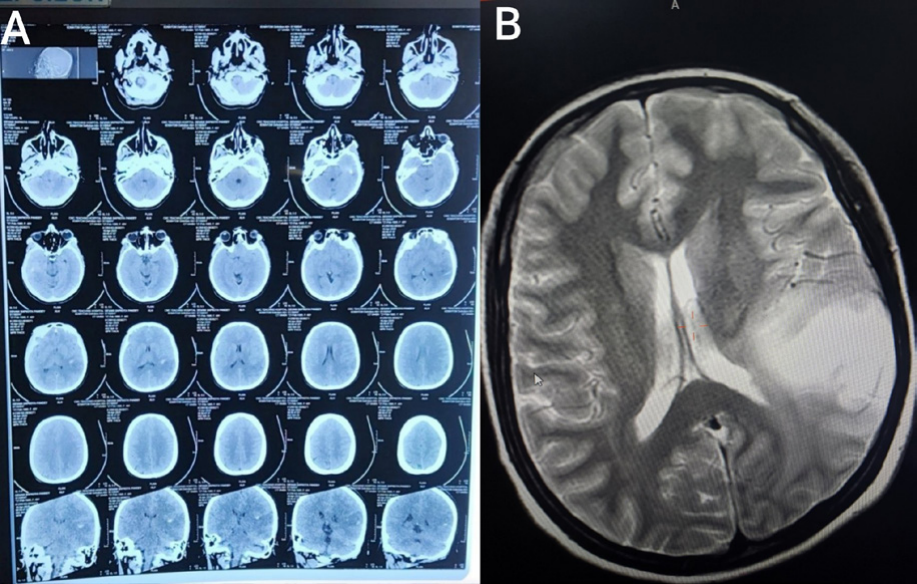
Pre-operative imaging studies. 1(A) Non contrast CT head. 1(B) MRI brain.
